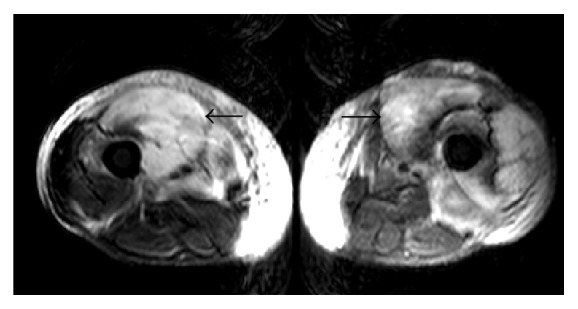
MRI of the thighs, coronal section, showing hyperintense signals on T2 weighted images bilaterally (black arrows).
